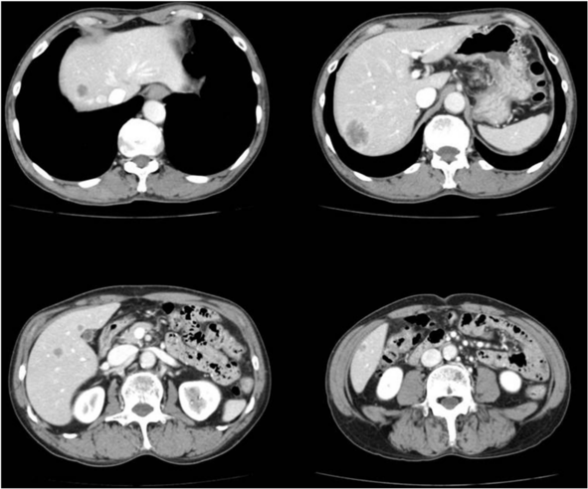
CT scan of this patient, showing multiple liver metastases in the liver.
